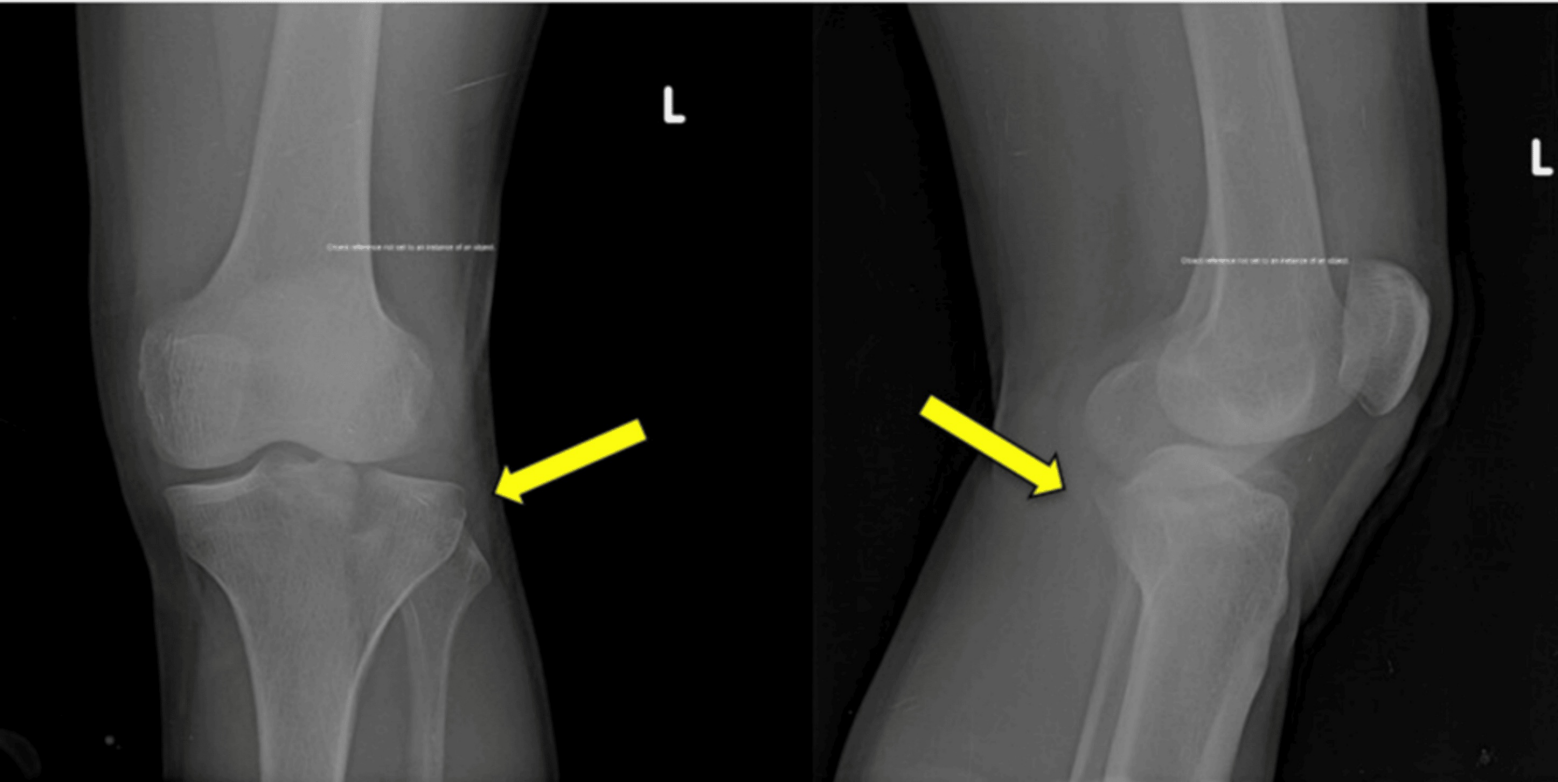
Preoperative anteroposterior and lateral radiographs demonstrating a proximal tibial fracture.
